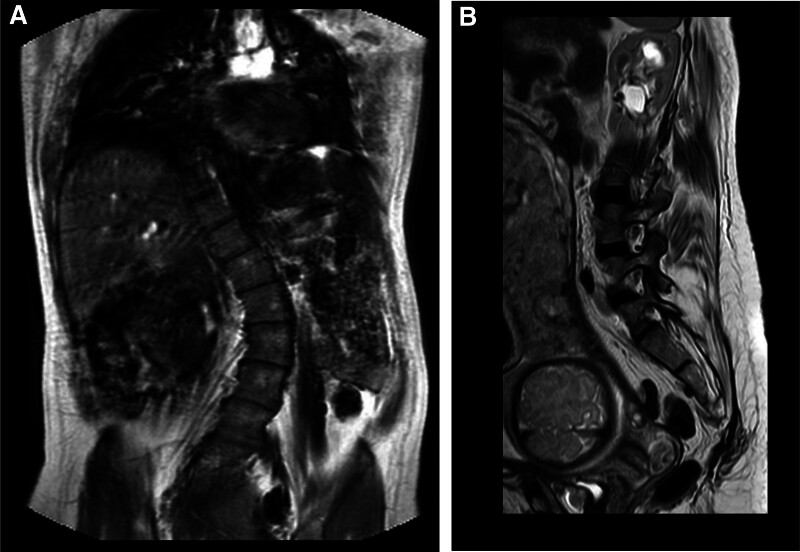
Thoracolumbar MRI. The thoracolumbar vertebrae exhibited an “S” shaped lateral curvature. The spinal cord within the spinal canal appeared smooth, with the conus medullaris positioned at the level of the L1 vertebra, and no space-occupying lesions were detected. There was a slight dilation in the left kidney along with a renal cyst. MRI = magnetic resonance imaging.
